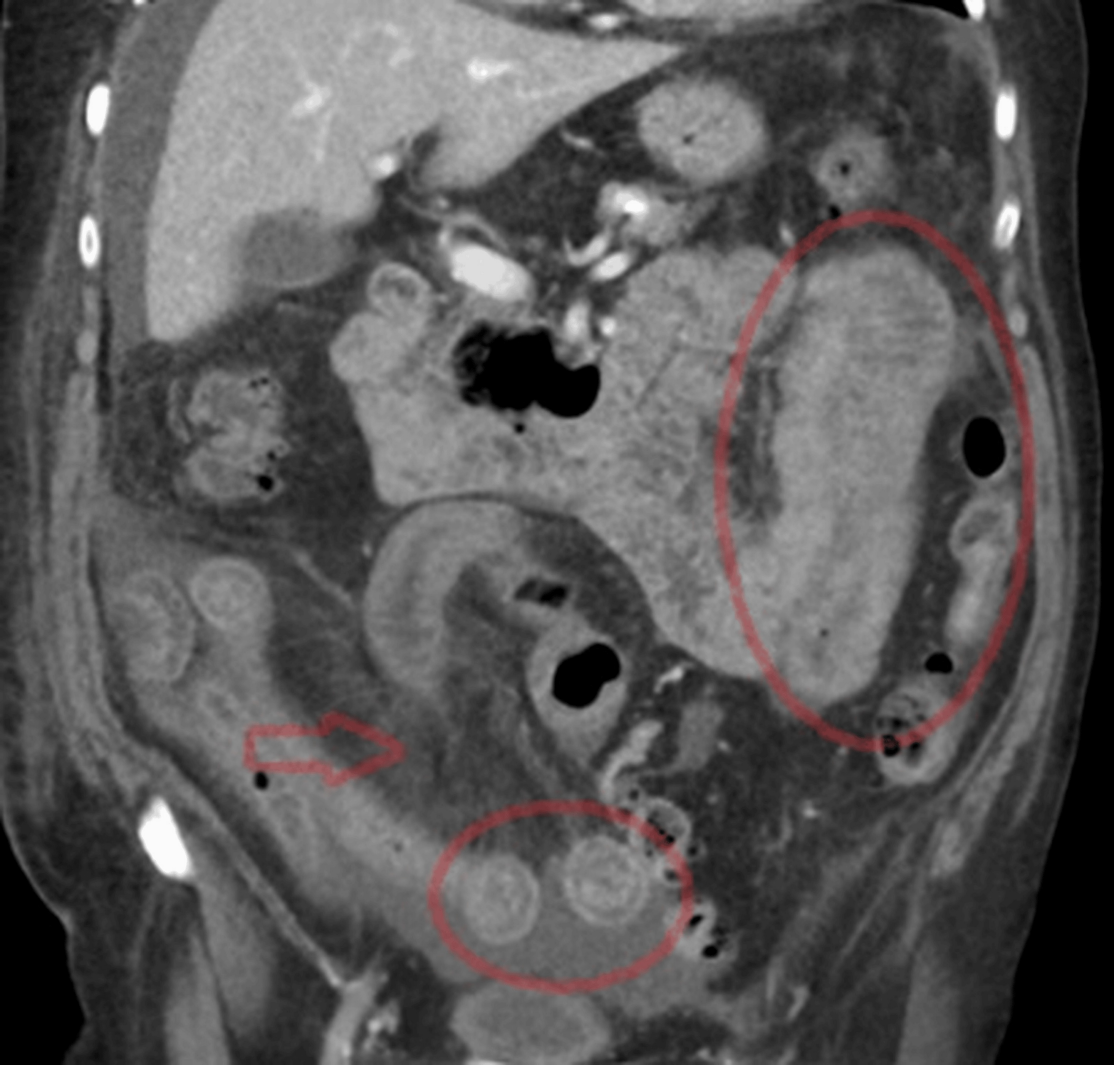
Coronal contrast-enhanced CT images showing concentric small bowel wall thickening with submucosal edema and stratified enhancement of the small bowel loops (red ellipses) with mesenteric comb sign (red arrow).
